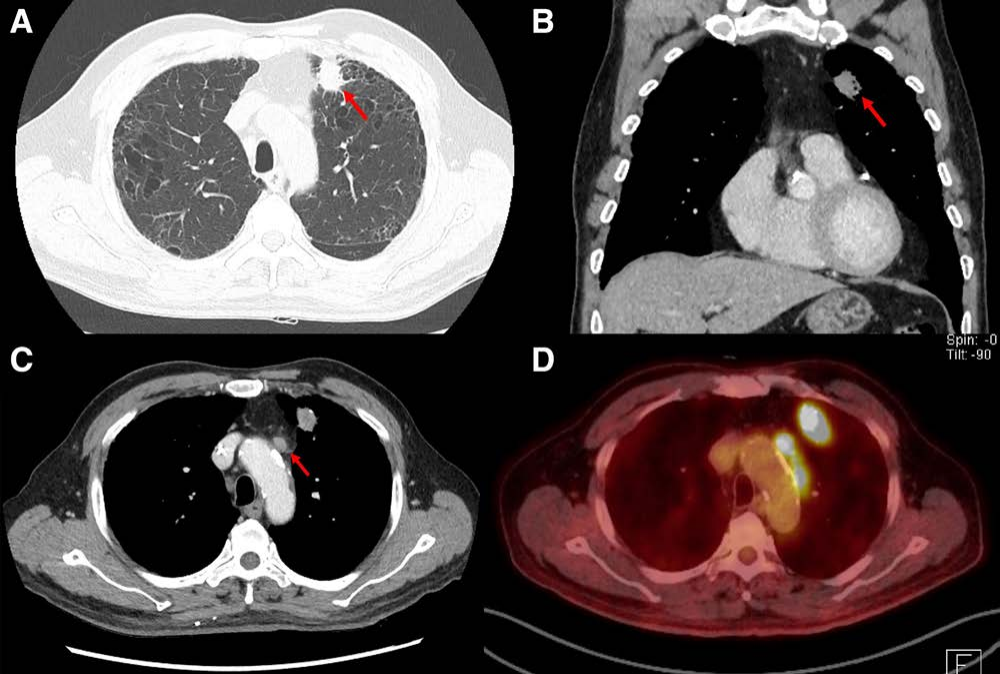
Initial diagnostic imaging data. (A) Computed tomography scan of the chest showing a 24-mm enhancing nodular lesion in the left upper lobe. (B) Multiplanar reconstruction on the coronal plane. (C) Para-aortic lymph node enlargement. (D) Positron emission tomography-computed tomographic image for para-aortic lymph node enlargement.
